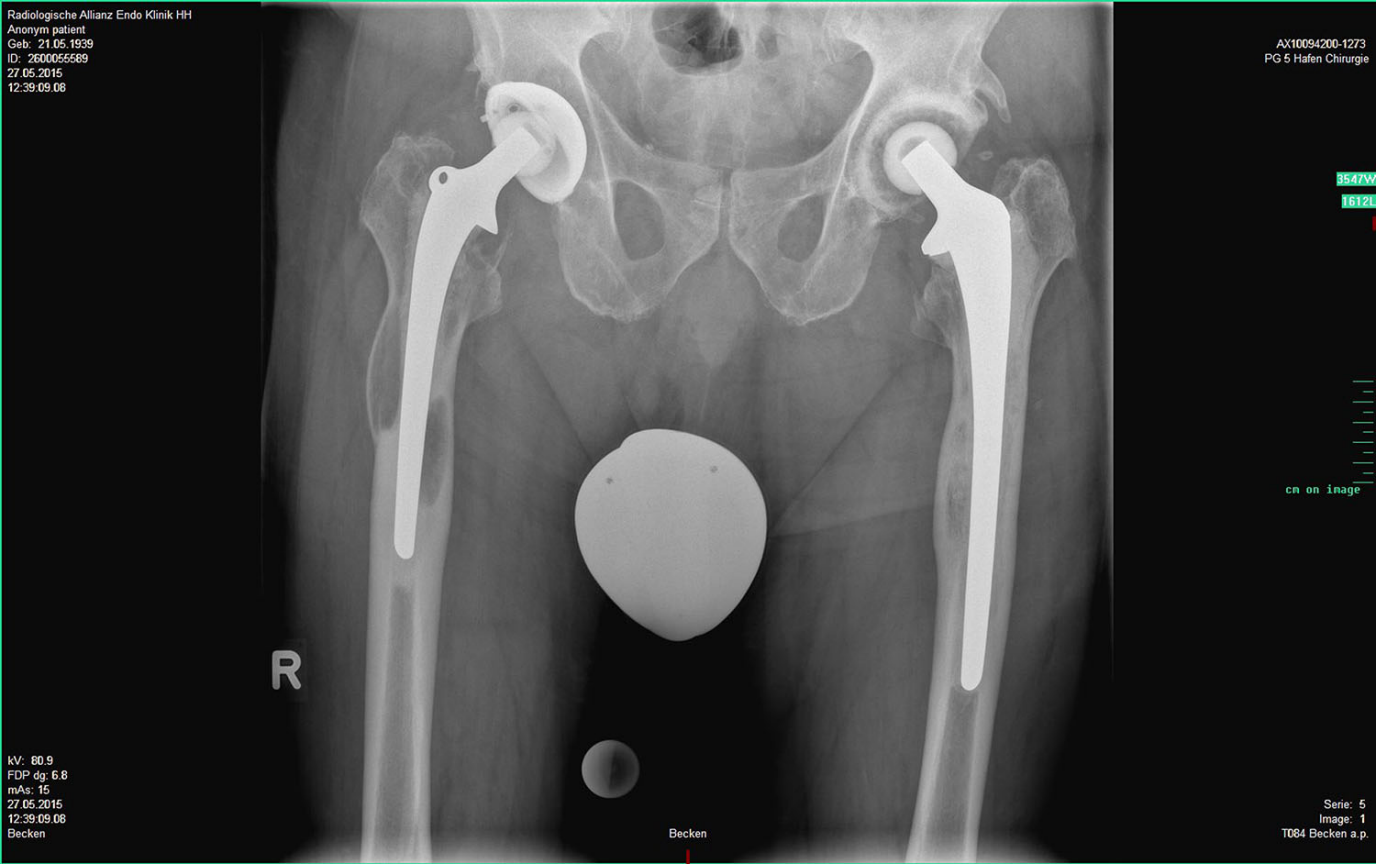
Preoperative AP radiograph of a patient with false-positive alpha-defensin value reveals polyethylene wear and osteolysis of the proximal femur at his right THA.
